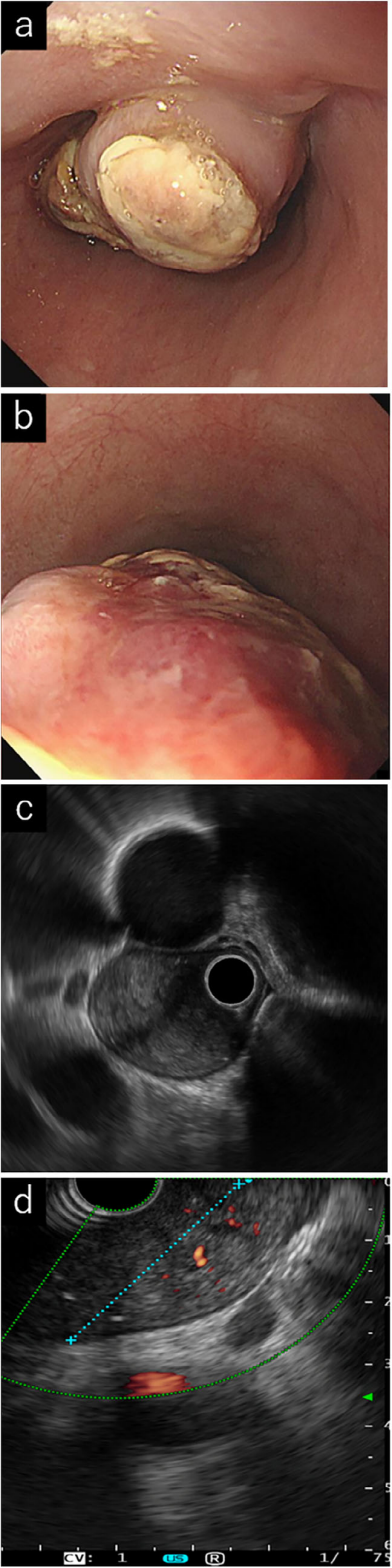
Endoscopic and endoscopic ultrasound findings after tumor enlargement to 5 cm. (a) Tumor with surface ulceration and a white coating, observed 2 years before endoscopic resection. (b) Reddening on the anal side of the tumor. (c) Endoscopic ultrasound image showing an intact muscle layer. (d) Doppler imaging demonstrating high echogenicity, suggesting internal bleeding and abundant blood flow.
